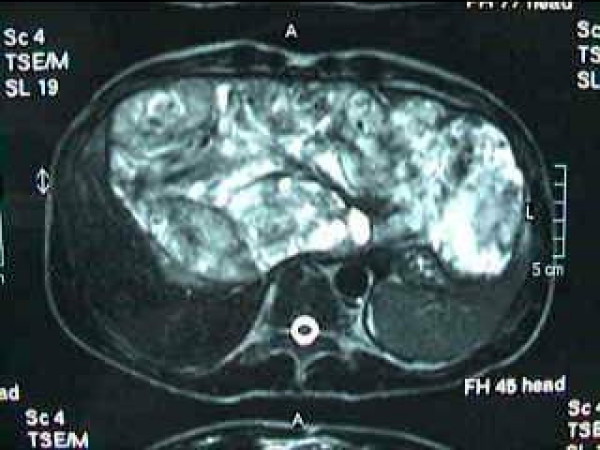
Magnetic Resonance Imaging (MRI) in T2-weighted and Short Time Inversion Recovery (STIR) sequences revealing hyperdense areas with intermixed hypodense septa.
